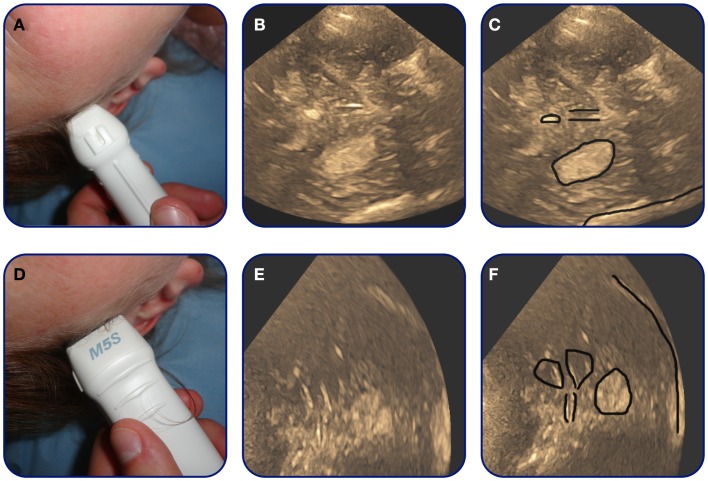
Transcranial duplex sonography-images of basal-ganglia hemorrhage. A patient with basal-ganglia ICH. On axial images (A,B) the hematoma can be observed as a hyperechogenic region in the middle of the picture. The rim of the hematoma is marked by black on (C). In addition on (C) the location of important midline landmark structures as the pineal gland and the third ventricle are marked. (D,E) Are coronal images. On (F) the lateral ventricles, the third ventricle, and the hematoma is marked.
